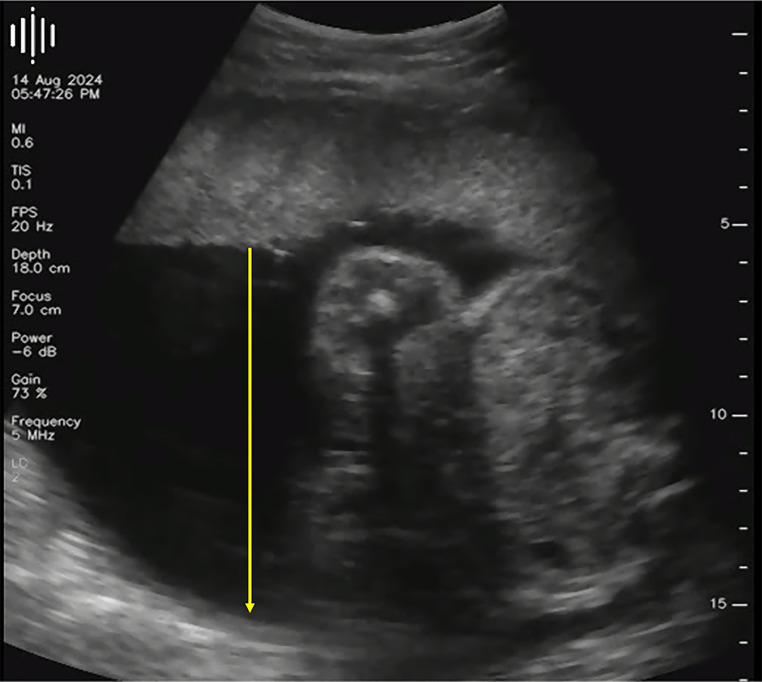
Participant using remote ultrasound device and demonstrating polyhydramnios measuring a maximum pool depth of 9.7 cm (yellow arrow).
